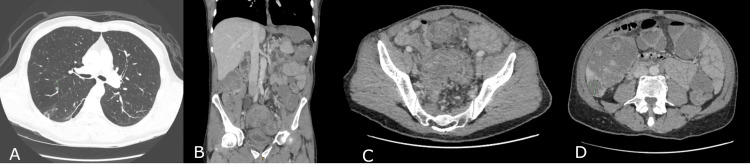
CT imaging with (A) axial CT slice demonstrating lung lesion, (B) coronal CT slice demonstrating sigmoid mass, (C) axial slice demonstrating sigmoid mass and (D) axial slice demonstrating hepatic metastasis
